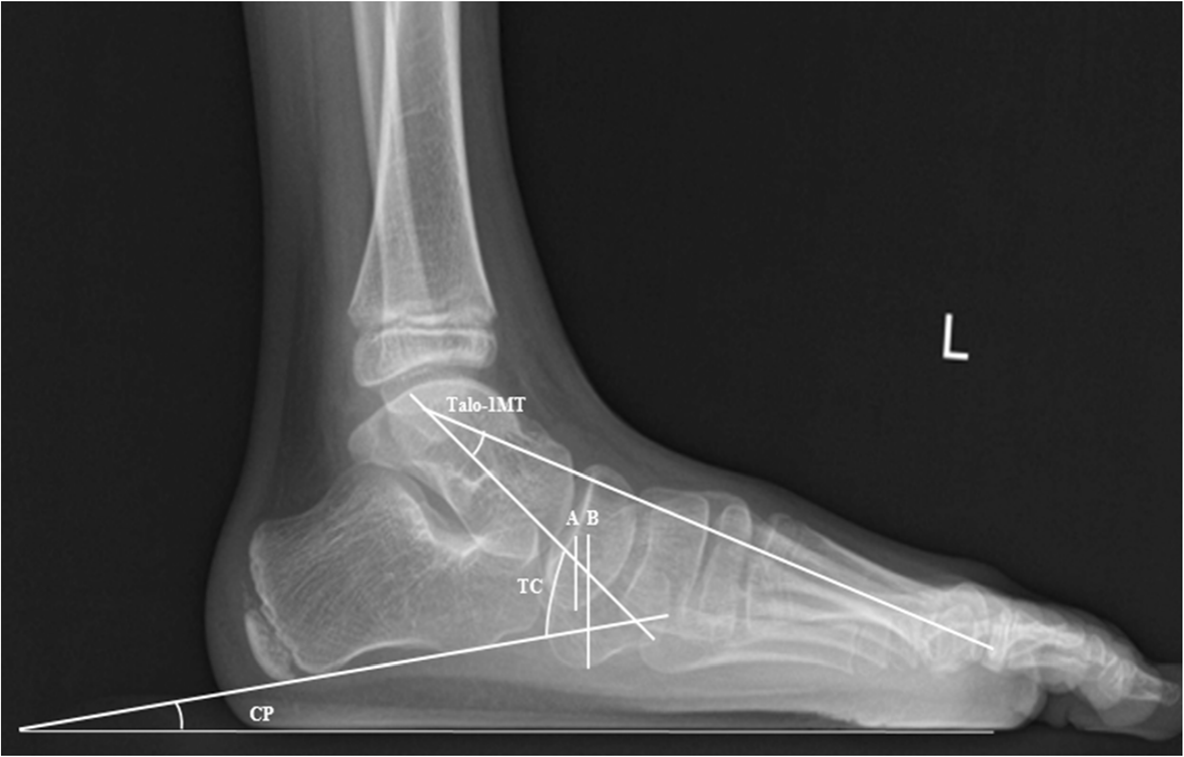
On weightbearing lateral (LAT) foot radiographs, the LAT talus-first metatarsal angle (LAT talo-1MT), calcaneal pitch angle (CP), LAT talocalcaneal angle (TC), and naviculocuboid (NC) overlap are measured
